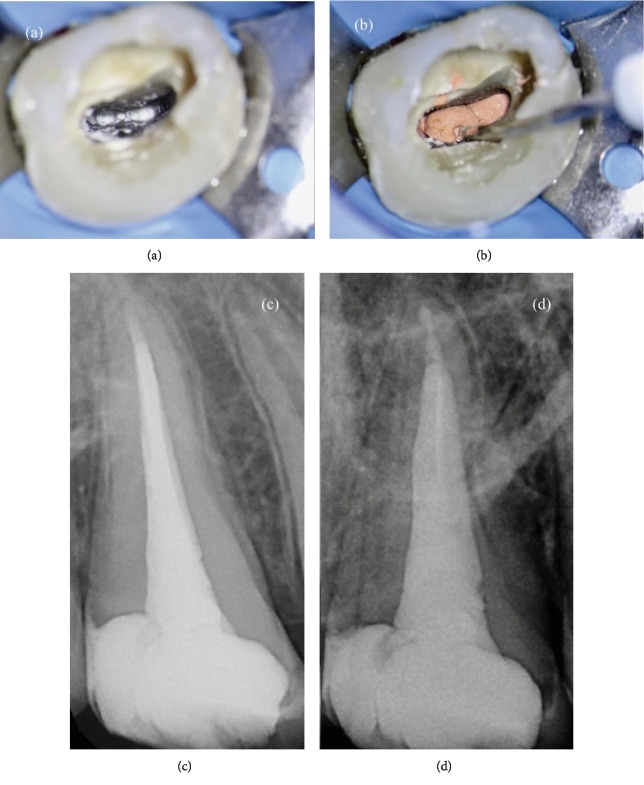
(a) Visualization of the apical plug. (b) Backfilling of the root canal system using thermoplastic gutta-percha. (c) Postoperative orthoradial X-ray. (d) Postoperative angulated X-ray.
